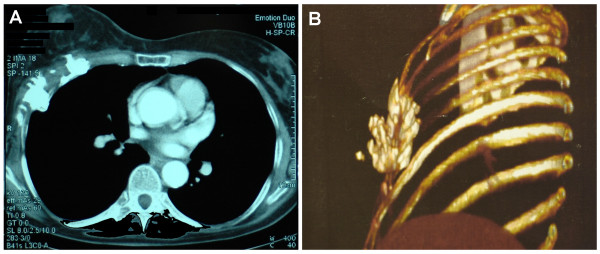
Pre-operative imaging studies of the tumor. Computed tomographic scan of the thorax (A) and respective 3-D image (B) exhibiting a large area of costal and muscular radionecrosis. The pleura was not affected.
